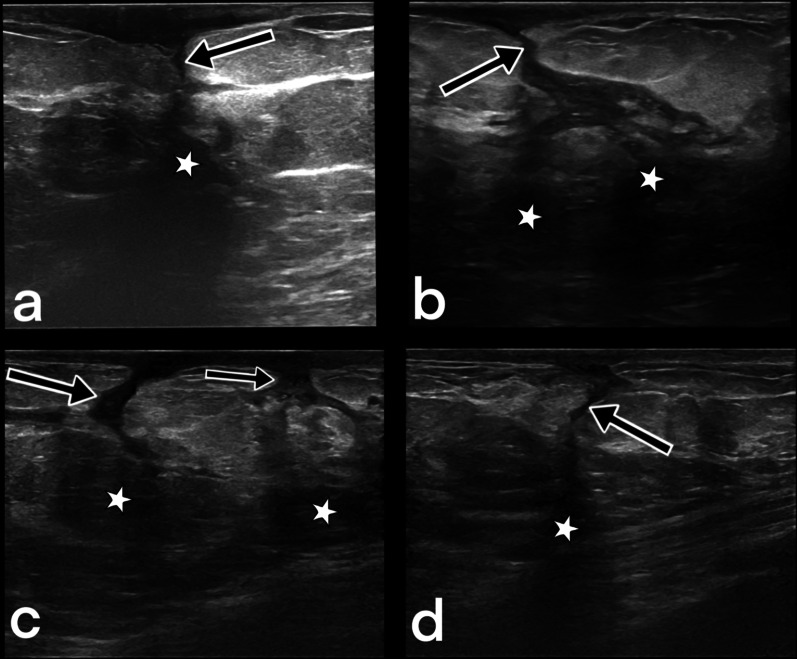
Ultrasound images from four different female patients (a–d). Sinus tracts (arrows) and related abscess formations (stars). BIRADS 4
